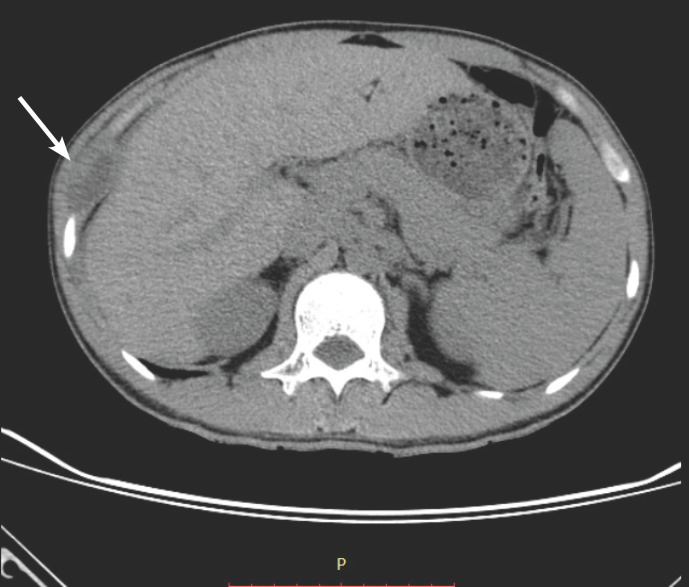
Scanner thoracique montrant la lésion en regard de la neuvième côte Chest CT scan showing the lesion over the ninth rib
